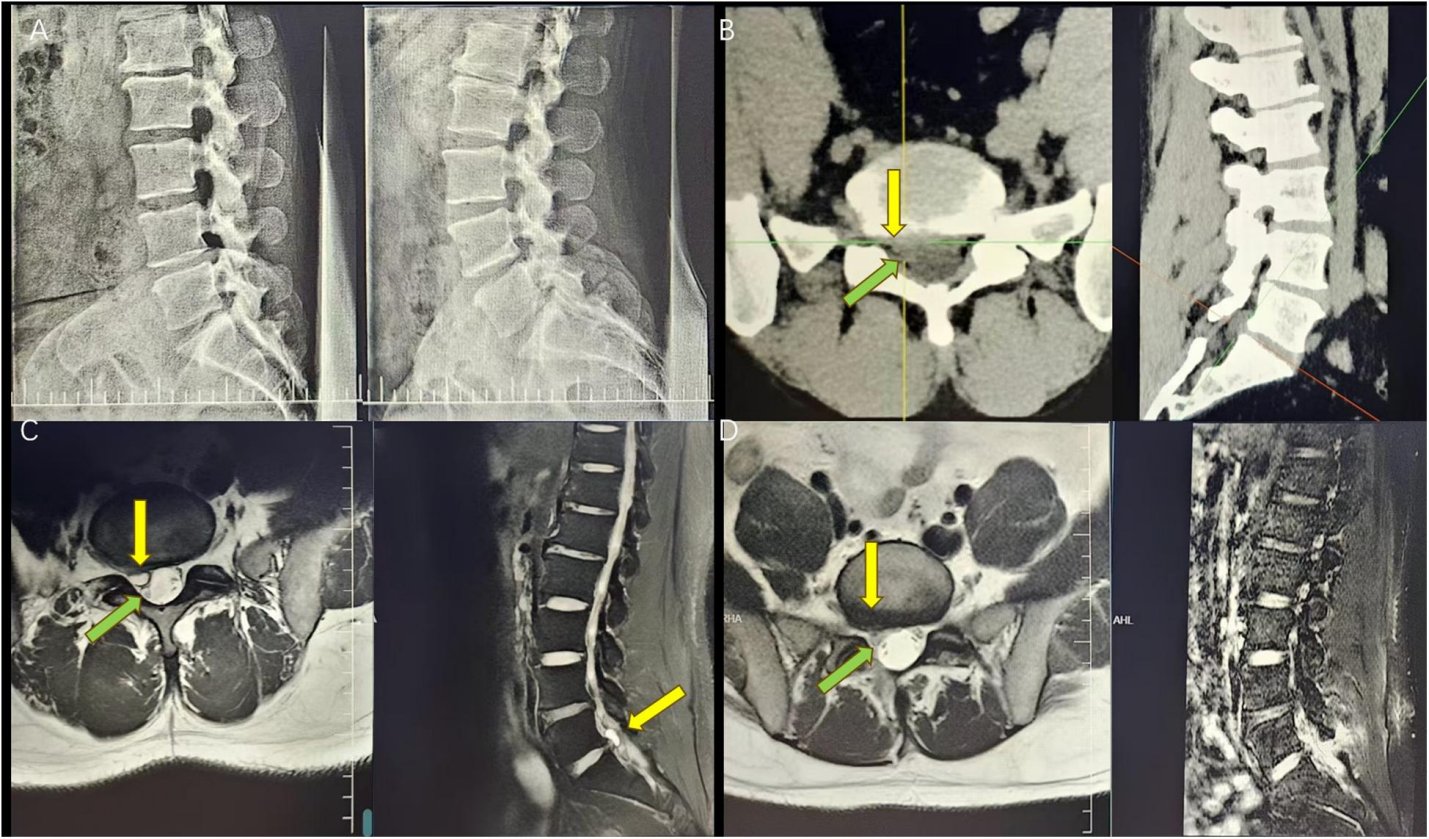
(A) Preoperative digital radiography (DR) showing normal intervertebral height and vertebral stability. (B) Preoperative CT showing a herniated disc on the right side of L5/S1 with the corresponding nerve root compression (the yellow arrow indicates the location of the herniated disc and the green arrow indicates the location of the compressed nerve root emanation). (C) Preoperative MRI showing a dense T1/T2 image with the corresponding nerve root compression (the yellow arrow indicates the location of the herniated disc and the green arrow indicates the location of the compressed nerve root emanation). (D) Postoperative MRI suggesting disappearance of the cyst and nerve root relaxation (the yellow arrow indicates the location of the original cyst and the green arrow shows nerve root relaxation).
